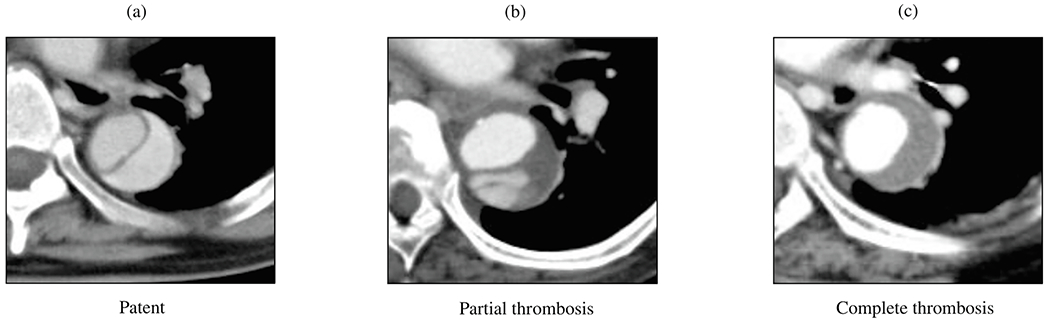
Representative enhanced CT images showing the status of the false lumen in aortic dissection: (a) patent, (b) partial thrombosis, and (c) complete thrombosis. Figure is adapted from Tanaka et al. [553] with permission from Elsevier.
